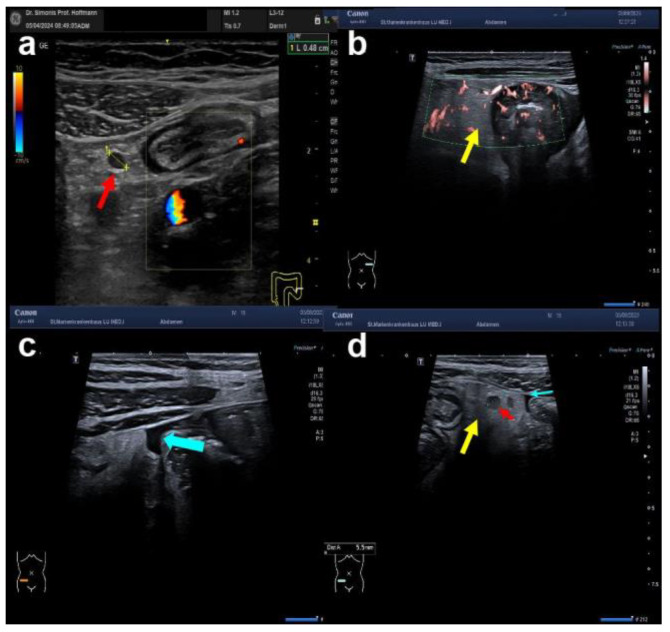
Additional extraintestinal ultrasound findings in IBD. (a) Shown is a round hypoechoic lymph node next to the thickened wall of the descending colon in hyperechoic tissue (fat wrapping) (yellow arrow). (b) The tissue surrounding the thickened strongly hypervascularised colonic wall (Limberg 4) is hyperechoic known as fat stranding, fat wrapping, or inflammatory fat (i-fat) (yellow arrow). (c) Ascites shown as an anechoic area indicated by a turquoise arrow. (d) Patient with Crohn’s disease showing all three additional extraintestinal signs, i.e., fat wrapping (yellow arrow), pericolonic lymph node (red arrow), and small amount of ascites (turquoise arrow).
